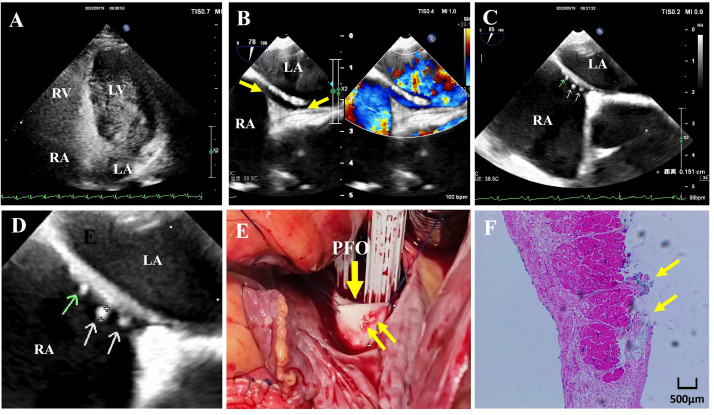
Typical case of *in situ* microthrombus in the atrial septum. (A) Saline Contrast Echocardiography showing an extremely large intracardiac right-to-left shunt (RLS) after the Valsalva maneuver. (B) Transesophageal echocardiography reveals a large patent foramen ovale (yellow arrows). (C,D) Transesophageal echocardiography displaying multi-tiny *in situ* thrombosis in the right atrial surface (green arrow and white arrows). (E) Intraoperative photo displaying the patent foramen ovale and *in situ* thrombosis (yellow arrows). (F) Pathological analysis verifying the existence of *in situ* thrombosis (yellow arrows), Scale bar = 500 μm. LA indicates left atrium; LV, left ventricle; RA, right atrium; RV, right ventricle; and PFO, Patent foramen ovale.
